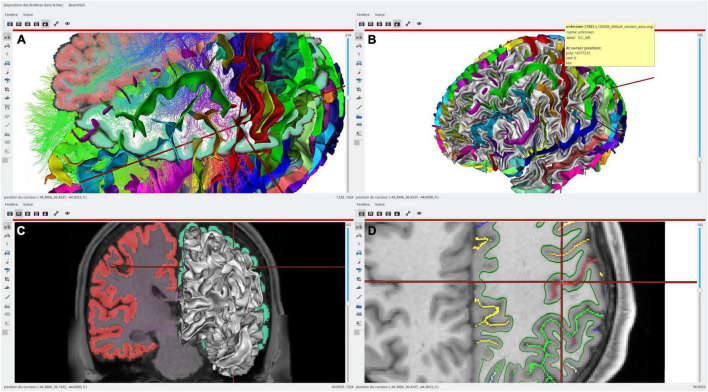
An example of single subject, multimodal data visualization using *Anatomist*. On this example, we have displayed: (A, top left) a skull-stripped T1 MRI slice and Freesurfer segmentation overlaid, a cortical sulci graph in 3D obtained using Morphologist, a subset of a tractography reconstructed by MRTrix, a clipped gray/white matters interface mesh with Desikan cortical regions obtained by Freesurfer; (B, top right) the brain gray/white interface mesh and cortical sulci; (C, bottom left) a skull-stripped T1 MRI slice and Freesurfer segmentation overlaid in coronal orientation and a left hemisphere gray/white mesh; (D, bottom right) a skull-stripped MRI and a 2D representation of cortical sulci in axial orientation, with the gray/white mesh intersection on this plane (green). The objects live in different coordinate systems for acquisition and/or processing reasons (different kinds of software are processing using different spaces), and affine transformations between them have been loaded in *Anatomist*. Object’s location and cursor position match in all views.
